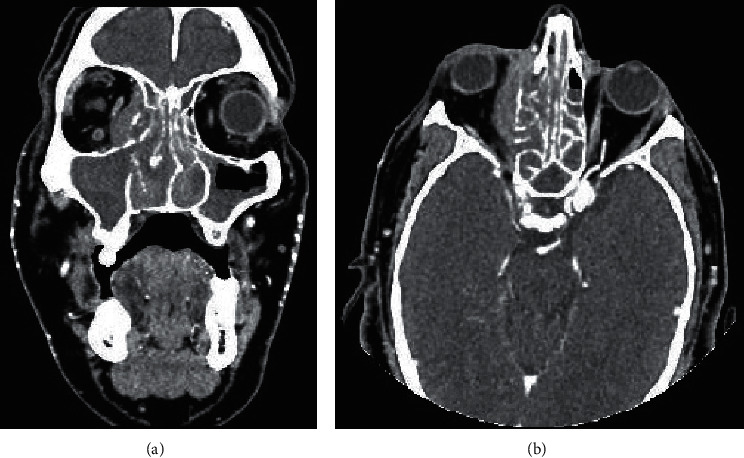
CT maxillofacial with IV contrast showing opacified ethmoid sinuses with bone destruction of the right lamina papyracea with soft-tissue extension into the right orbit. (a) Coronal view; (b) axial view.
